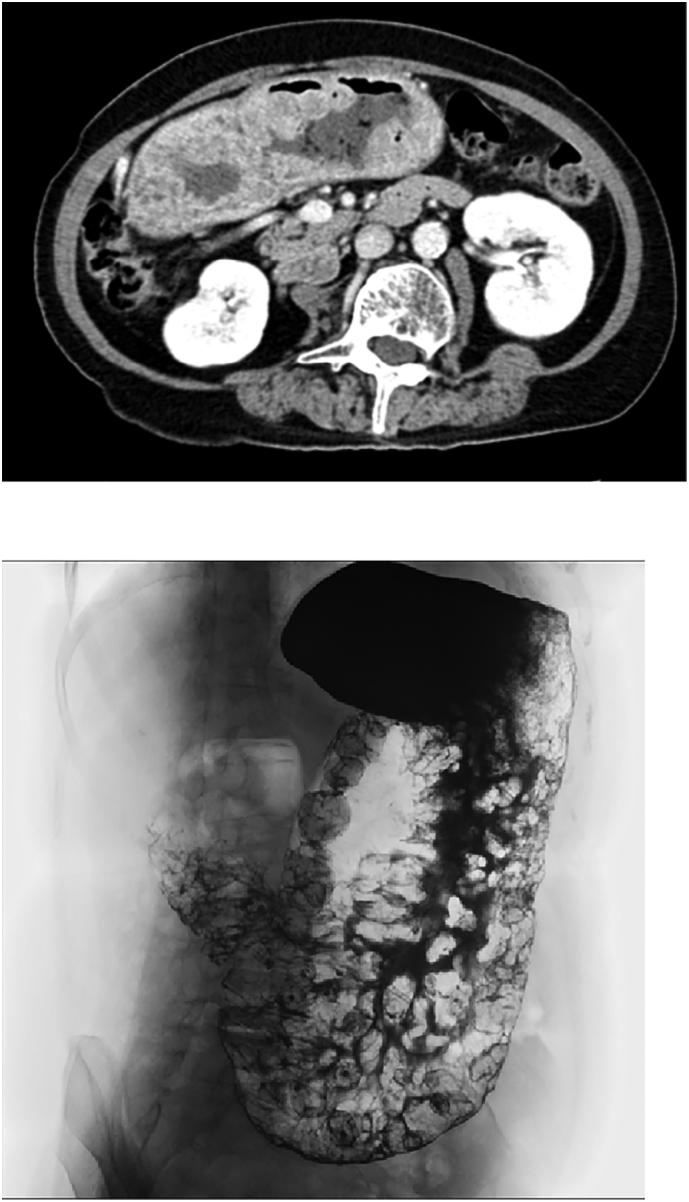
Enhanced computed tomography showed gastric wall thickening and multiple gastric polyps without lymphadenopathy or distant metastasis (A). Upper gastrointestinal series revealed that multiple polypoid lesions were widespread throughout the entire stomach except for the lesser curvature and the fundus (B).
